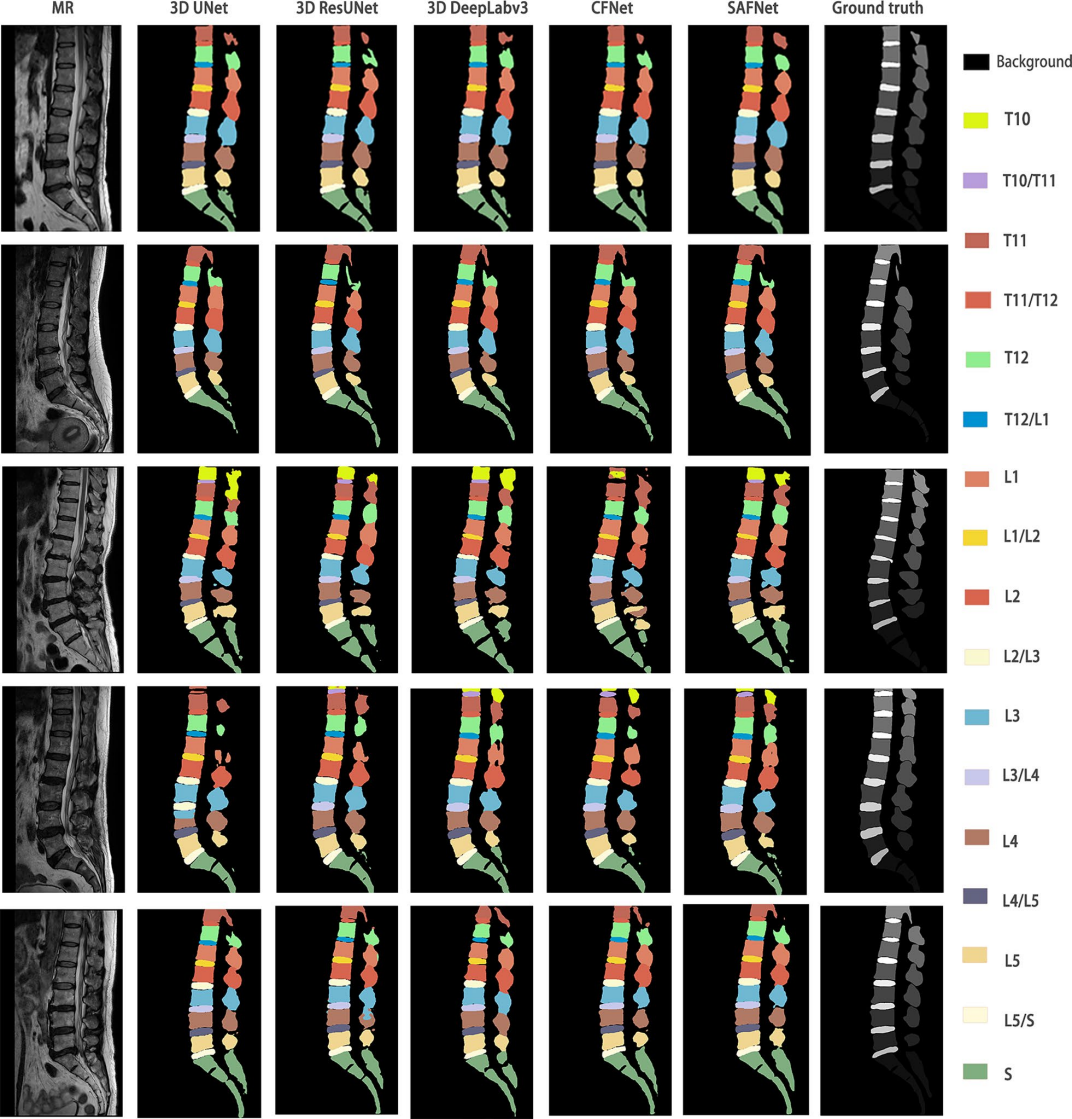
SAFNet has the ability to enhance the differentiation of each vertebra and intervertebral disc. SAFNet is designed to extract and learn semantic features from the input medical images, which can effectively distinguish different anatomical structures within the spine. Each row in the figure represents the middle sagittal slice of a subject. (SAFNet denotes scene aware fusion network; BG denotes background; MRI denote magnetic resonance imaging)
